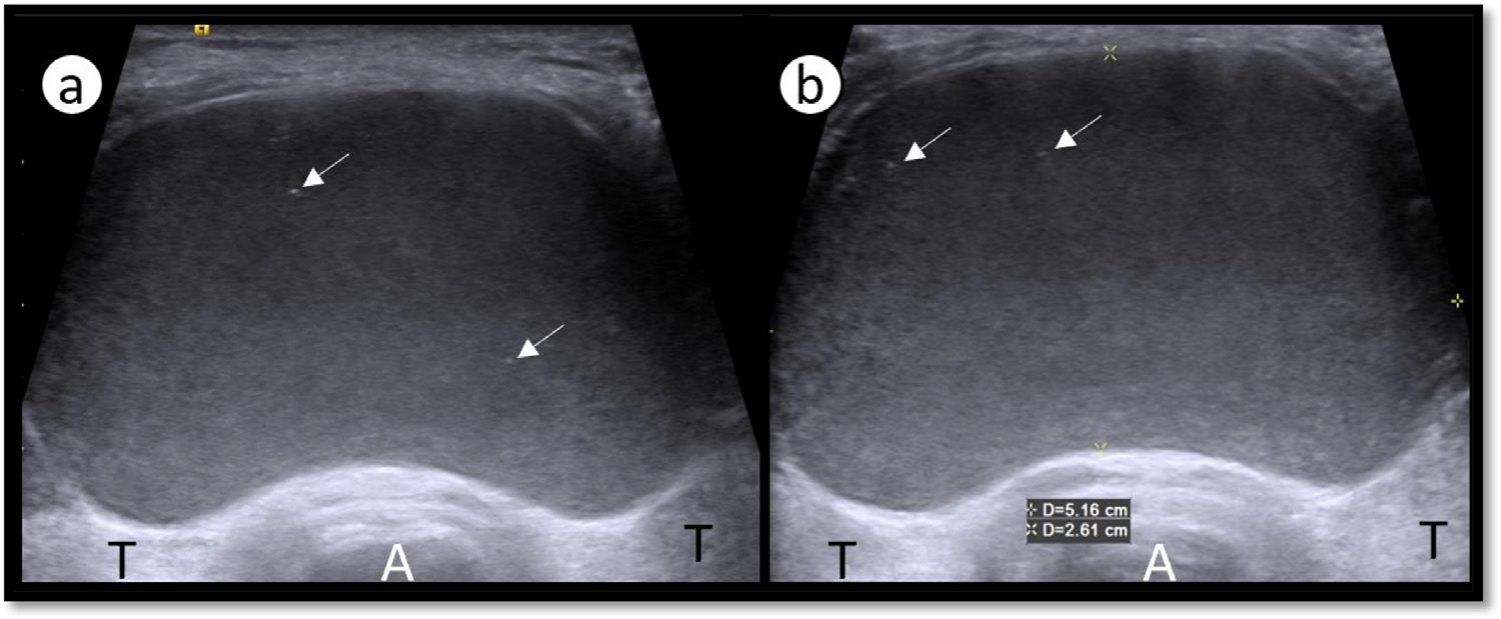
(a,b): Ultrasound neck showing a large cyst having homogenously low echogenic content with few tiny echogenic foci showing no comet tail artifact (arrows). Thyroid lobes (T) and airway (A).
